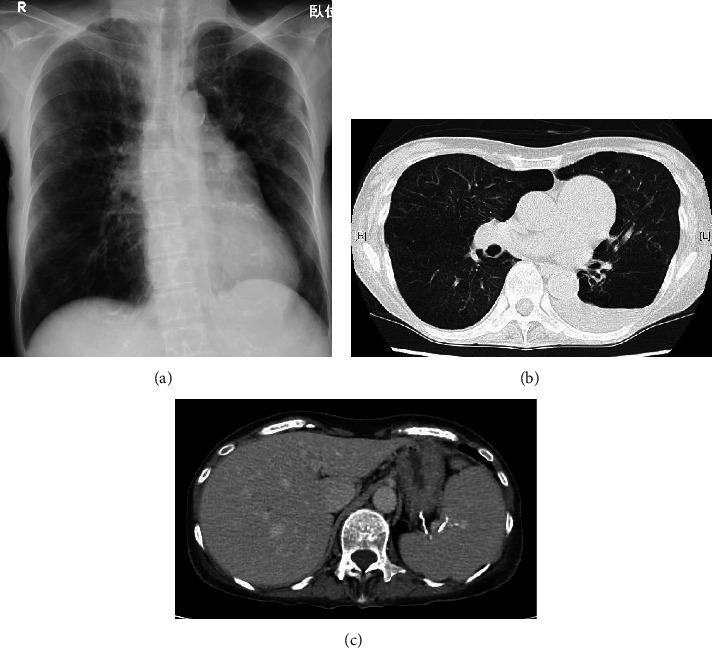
Chest X-ray revealing cardiac enlargement and emphysematous shadows in both the lungs (a). Computed tomography revealing emphysematous shadows in almost all lung fields, left-side pleural effusion (b), and splenomegaly (c).
